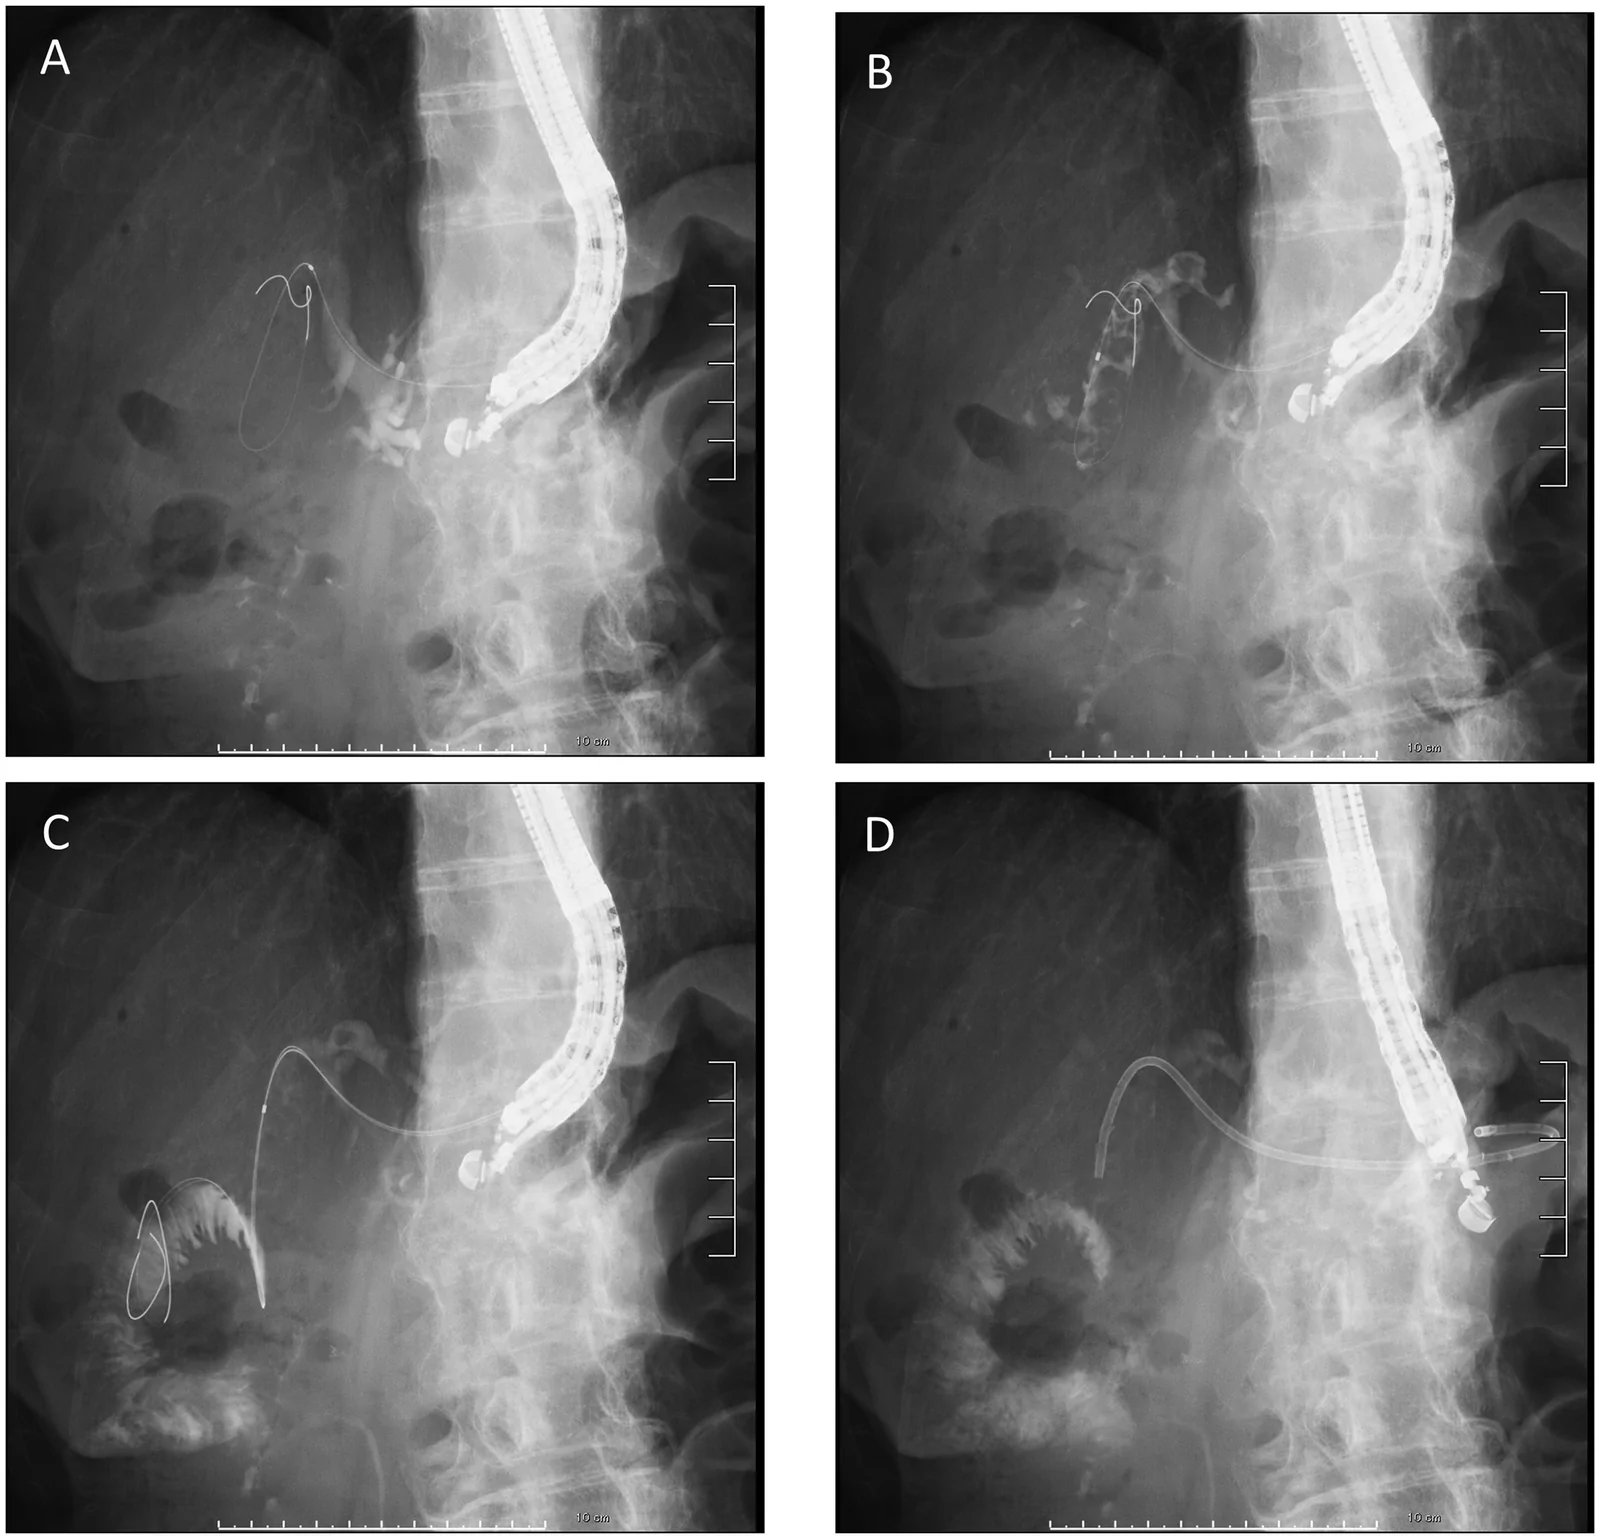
Endoscopic ultrasound (EUS)-guided hepaticogastrostomy using a 22-G needle and a novel double-lumen catheter. (A) Puncture of the B3 intrahepatic bile duct using a 22-G needle under EUS guidance, followed by contrast medium injection and placement of a 0.018-inch guidewire in the bile duct. (B) Advancement of the novel double-lumen catheter over a 0.018-inch guidewire without prior tract dilation. (C) Placement of an additional 0.025-inch guidewire through the novel double-lumen catheter using the double-guidewire technique. (D) Placement of a 7-Fr plastic stent in the bile duct without additional dilation.
